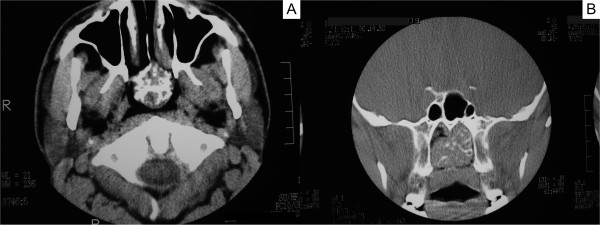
(A and B) Axial and coronal computed tomography sections. No adipose tissue or cystic components were seen on the computed tomography (CT) scan.
